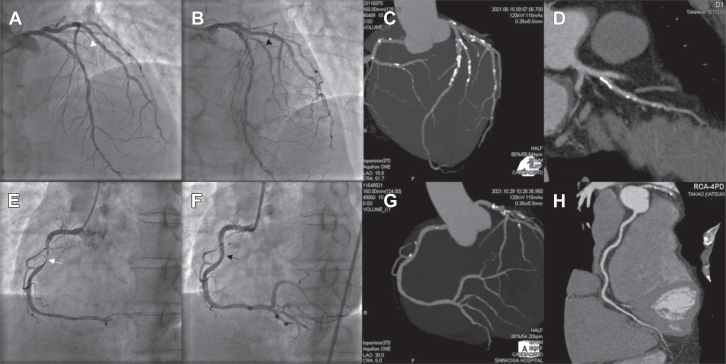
Typical example of the 2-year follow-up of RYUSEI drug-coated balloon (DCB) treatment. (A) Initial coronary angiogram of a man in his 60s with ST-segment elevation myocardial infarction (STEMI); angiogram demonstrates 1st diagonal occlusion (arrowhead △). (B) Final angiogram after DCB (2.5 mm) dilation demonstrates a stent-like result (arrowhead ▲). (C) and (D) Coronary computed tomography angiography (CCTA) at the 2-year follow-up reveals mild stenosis at the culprit lesion. (E) Initial coronary angiogram of a man in his 40s with (STEMI); angiogram demonstrates proximal right coronary artery severe stenosis (white arrow). (F) Final angiogram after DCB (3.5 mm) dilation demonstrates a stent-like result (black arrow). (G) and (H) CCTA at the 2-year follow-up reveals mild stenosis at the culprit lesion.
